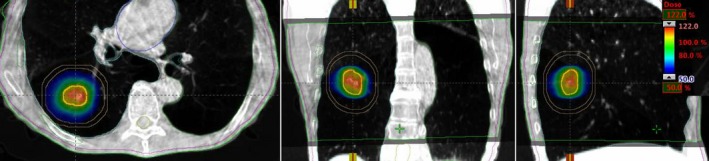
Axial, coronal, and sagittal views of CBCT images (see inset) co‐registered with planning CT images (see back at coronal and sagittal views) used for image‐guided SBRT treatment. In addition to anatomical landmarks, the planned dose cloud was superimposed. CBCT images were acquired in free breathing with abdominal compression, and soft‐tissue three‐dimensional (3D) matching was performed manually. SBRT, stereotactic body radiotherapy.
